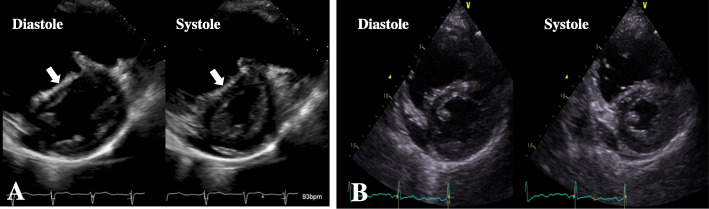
Transthoracic echocardiography before (a) and after (b) balloon pulmonary angioplasty. Flattening interventricular septum (arrow) was improved after balloon pulmonary angioplasty
